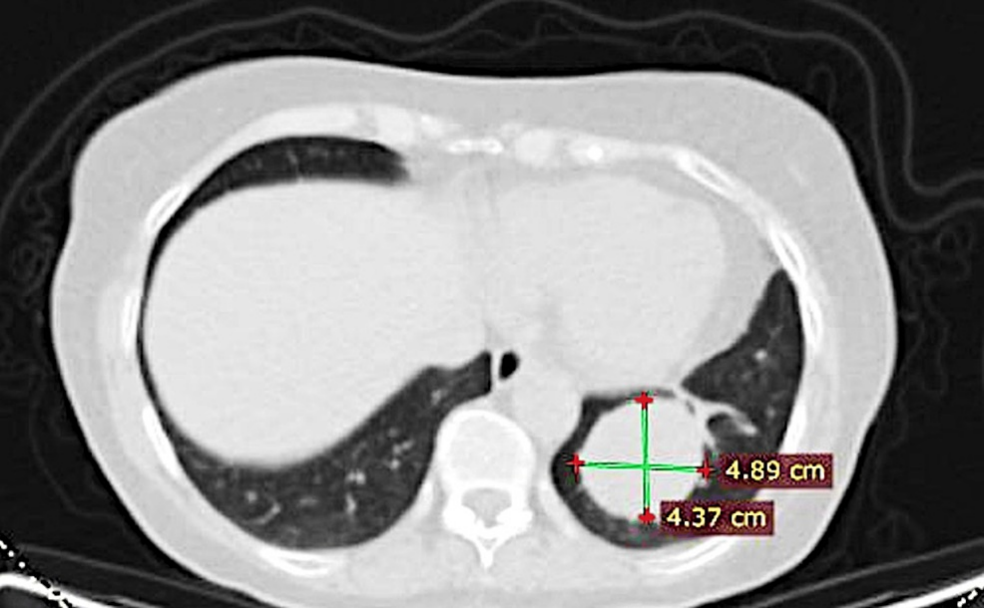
CT scan showing the SFT in the left lower lobe in 2013 CT: computed tomography; SFT: solitary fibrous tumor
